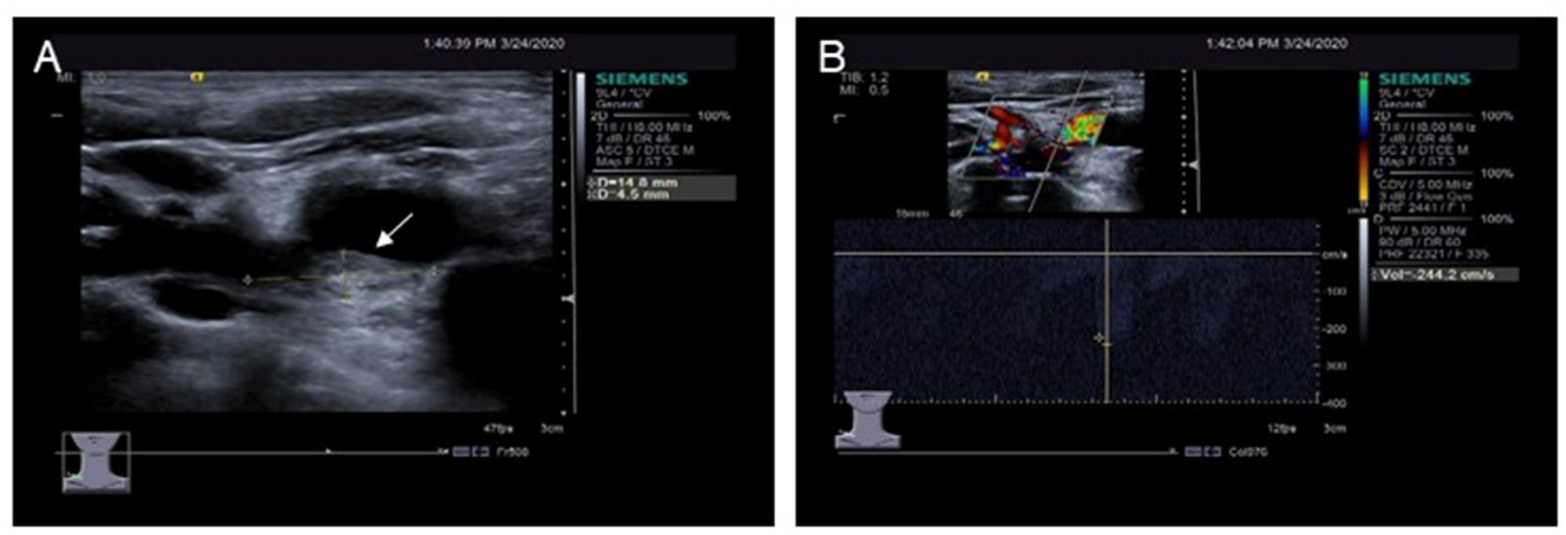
Ultrasound examination of the right clavicular artery. (A) A plaque approximately 4.5 mm × 14.8 mm in size is seen in the initial segment of the right clavicular artery. (B) Blood flow speeds up in the plaques of the right clavicular artery.
